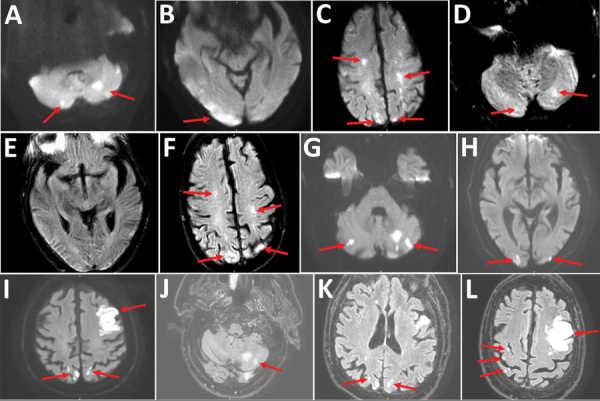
Cerebral magnetic resonance image (MRI) showing acute ischemic stroke in multiple vascular areas of 2 coronavirus disease patients, France. A–F) Patient 1. Diffusion weighted imaging (DWI) showed hyperintensive lesions of bilateral cerebellar hemispheres (arrows, A), right occipital cortex (arrows, B), bilateral centrum semiovale and bilateral parietal cortex (arrows, C). A part of the lesions are already hyperintensive in FLAIR (fluid-attenuated inversion recovery) sequences (arrows, D, F). Normal FLAIR sequence of the right occipital cortex; early stroke MRI (E). MRI quality is reduced because of dental artifact. G–L) Patient 2. Cerebral MRI showed multiple small ischemic infarctions with hyperintensive lesions (arrows) in bilateral cerebellar hemispheres (DWI [G], FLAIR [J; only left hemisphere]), bilateral occipital cortex (DWI [H], FLAIR [K]), main infarction in the left frontal lobe and small biparietal infarctions (DWI [I], FLAIR [L]).
